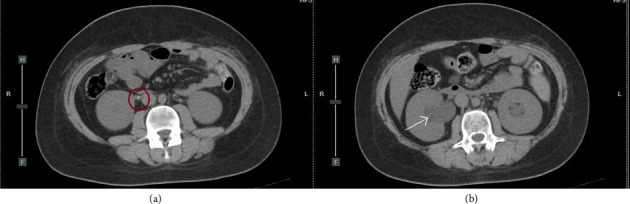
(a) CT scan. The circle indicates the stone located in the right ureter. (b) CT scan. The arrow indicates the dilation of the right pelvi.
